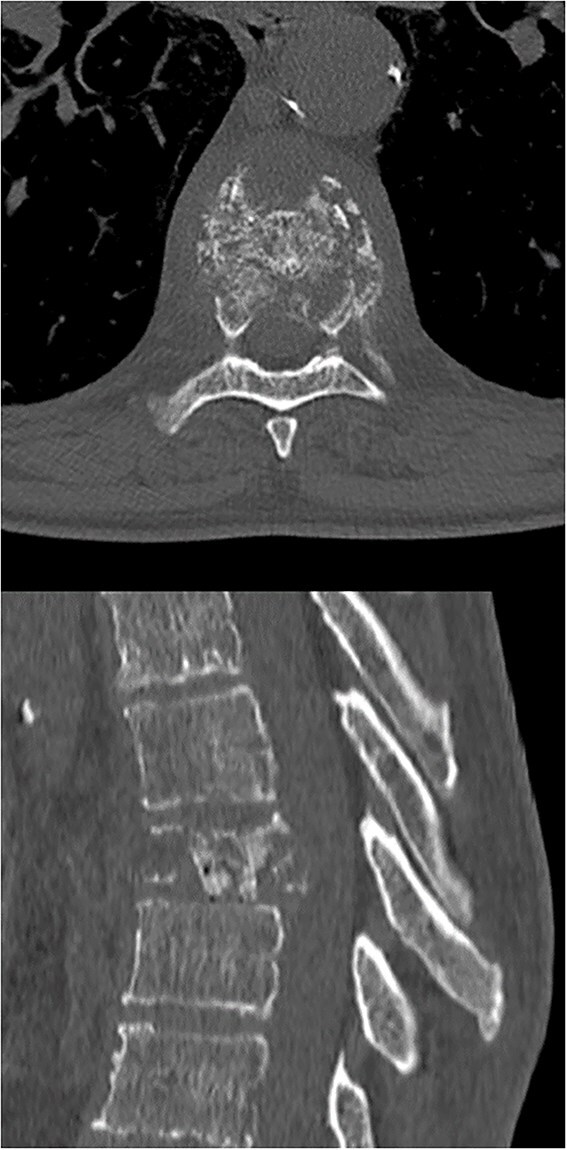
A computed tomography scan with axial (upper image) and sagittal plane (lower image) reconstructions with ‘bone’ filter revealing a compression fracture of the T8 vertebral body. The scan showed multiple fracture lines with a mixed lytic-sclerotic tumor component, with the lytic aspect being more prominent in the anterior portion of the vertebral body. There was also mild posterior wall prominence, but without significant stenosis of the vertebral canal.
